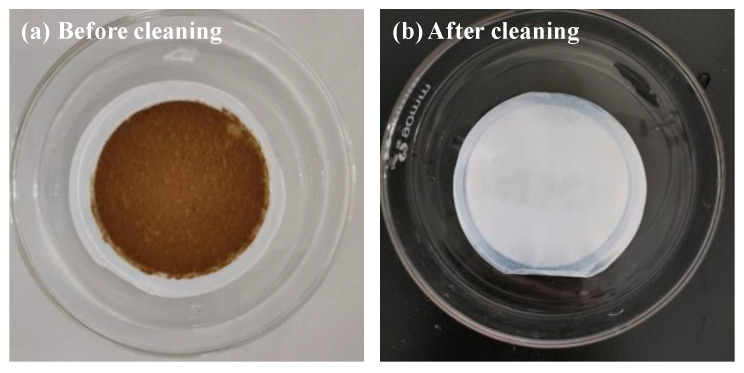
Typical photographic images of the ENMs with recycled and concentrated EPS (a) before and (b) after ultrasonic cleaning.
